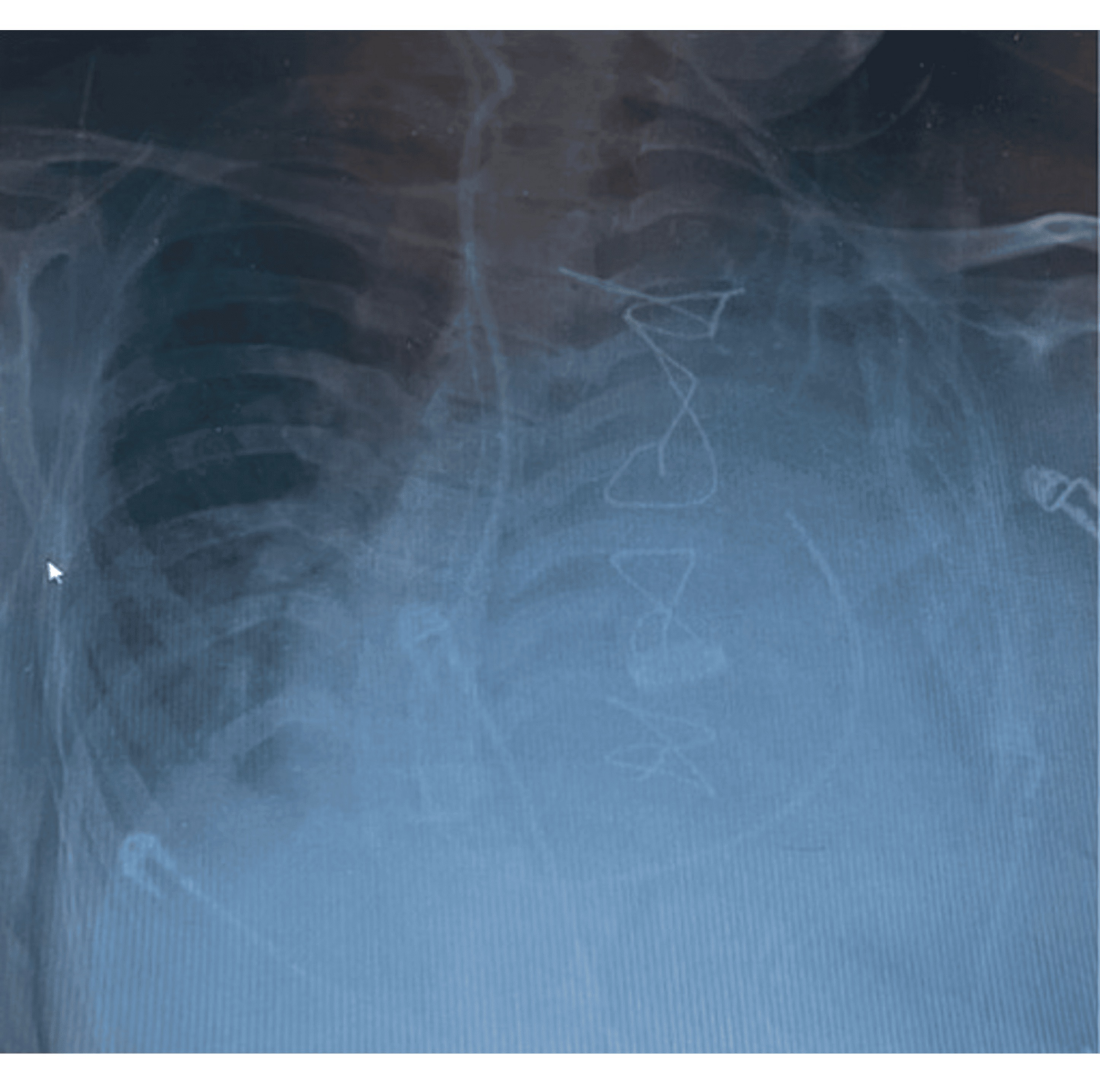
Postoperative chest X-ray showing respiratory failure. The image shows bilateral patchy air-space opacities predominantly involving the lower lung zones, more pronounced on the right side, with reduced lung volumes, consistent with postoperative pulmonary complications contributing to impaired gas exchange.
